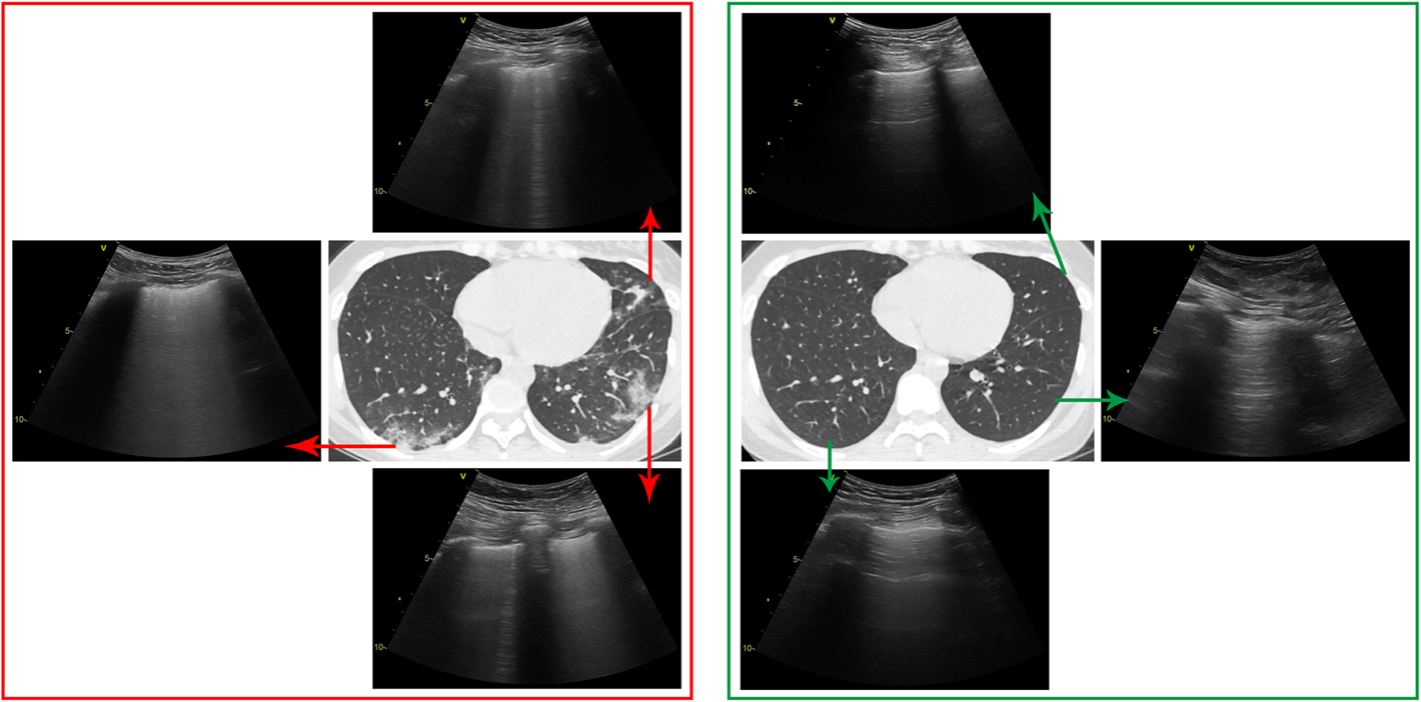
Lung ultrasound follow-up of a pregnant patient. The red frame on the left shows the lung images of the patient on admission; both ultrasound and CT showed obvious lesions in the peripheral zone. After 34 days of treatment, the lesions were completely absorbed at discharge, and both ultrasound and CT images were normal, as shown in the green frame on the right
